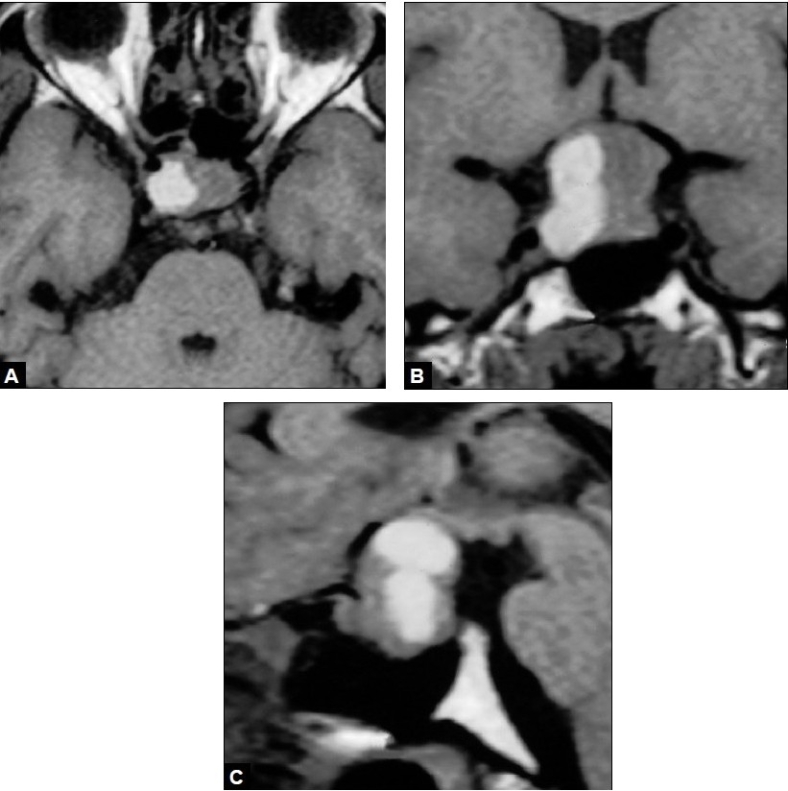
A: MRI Brain T1W (axial) images showing pituitary tumor with intratumoral hemorrhage; B:MRI Brain T1W (Coronal) images showing lelevation of optic chiasma; C: MRI Brain T1W (sagittal) images showing pituitary tumor with intra and suprasellar extension with pituitary aoplexy
